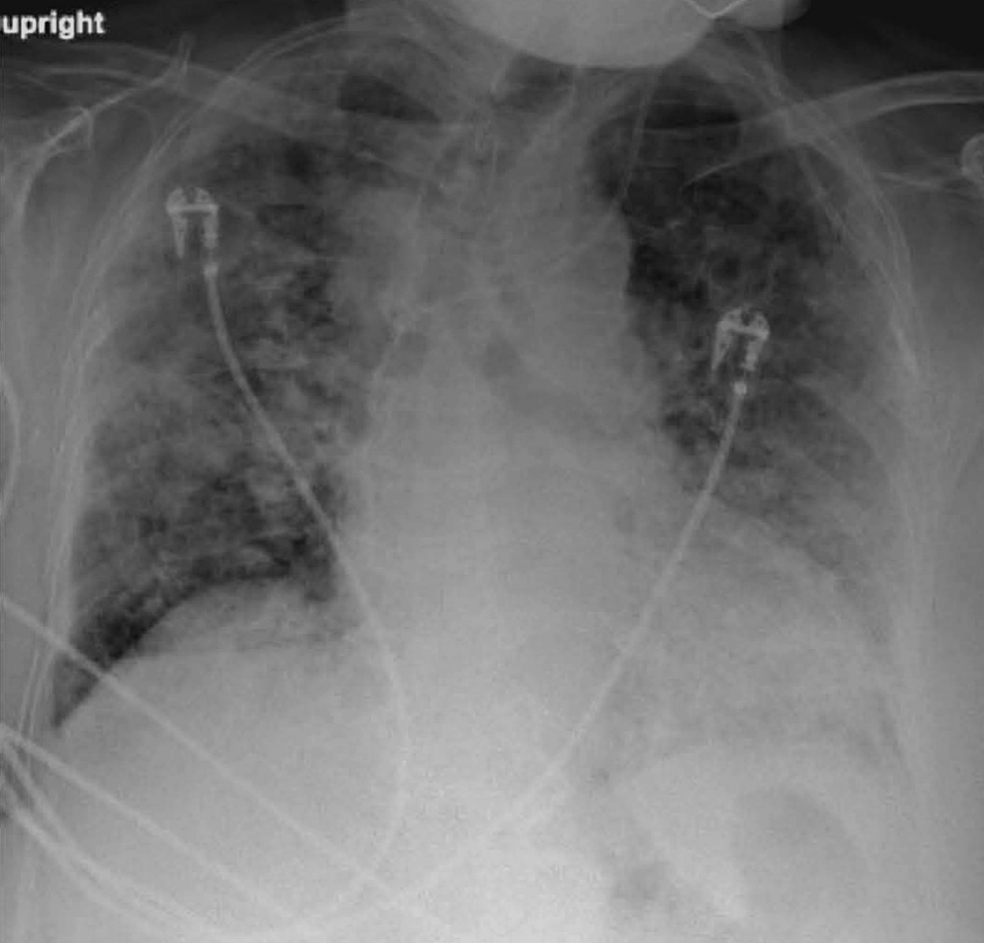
Admission chest X-ray showed bilateral parenchymal opacification
